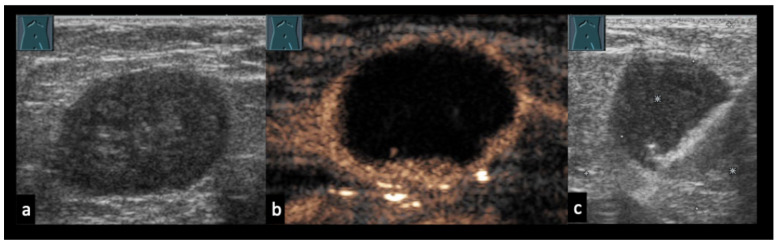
64-year-old female patient with vulvar carcinoma and a hypoechoic lymph node on B-mode ultrasound (a). (b) On CEUS at 20 s, the lymph node shows only peripheral rim enhancement. (c) Fine-needle aspiration revealed a basaloid squamous cell carcinoma with HPV association.
